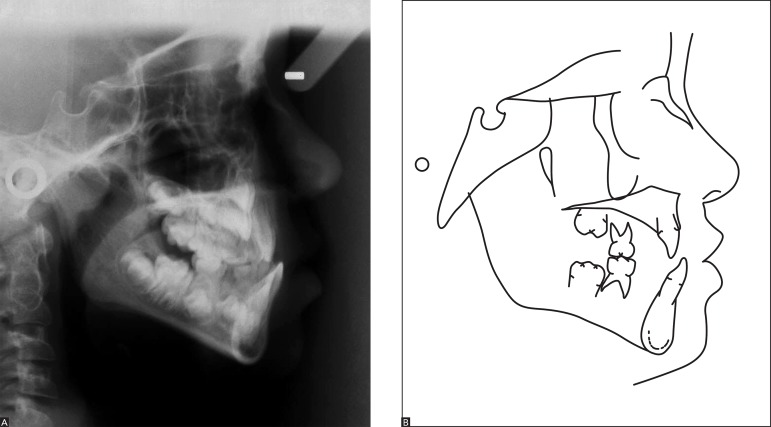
Initial profile cephalometric radiograph (A) and cephalometric
